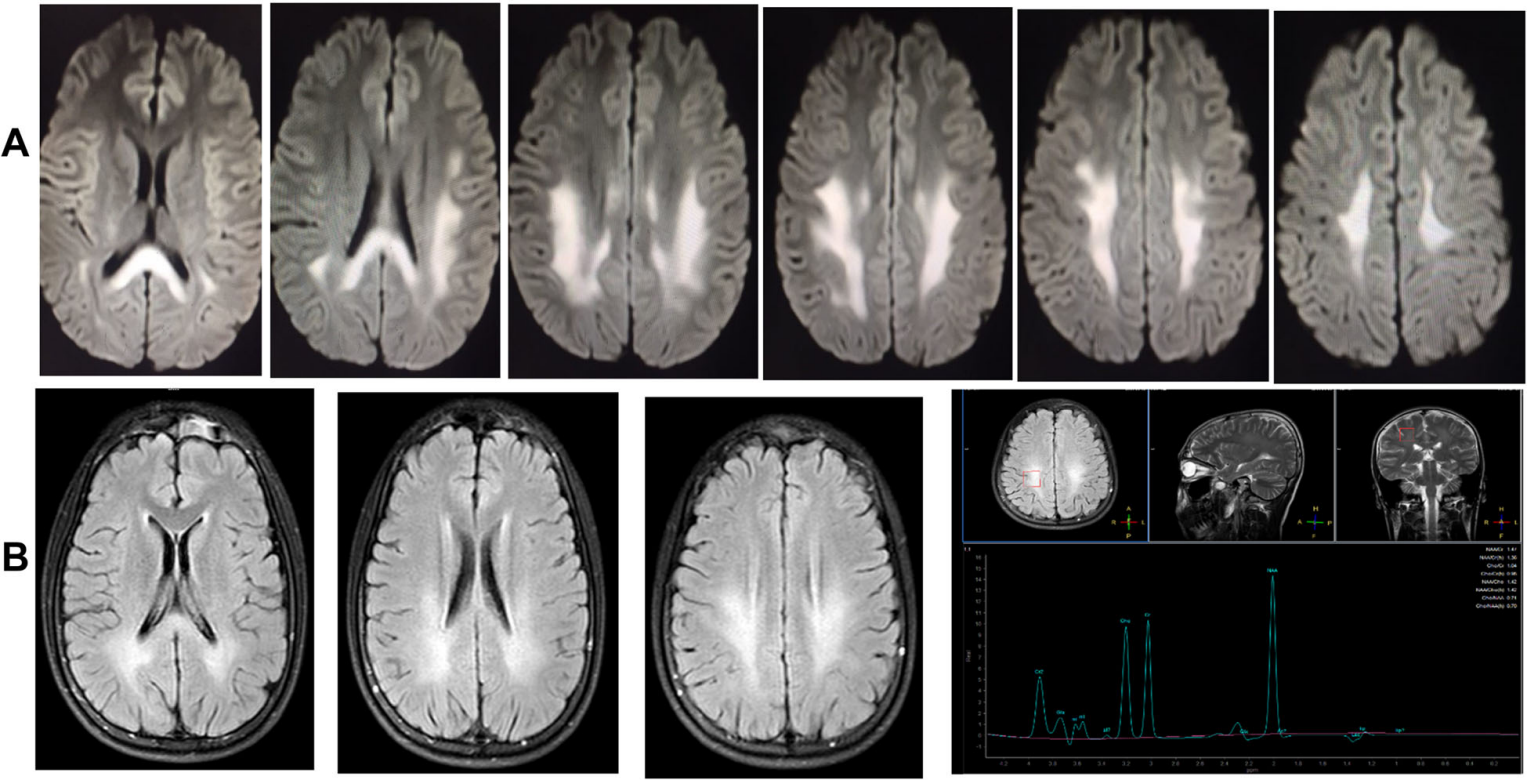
Brain magnetic resonance images of patient 1. a: MRI on 24 July 2018: Diffuse white matter lesions in posterior subcortical areas and the splenium of the corpus callosum. MRA was normal. b: MRI on 6 August 2018: the demyelinating changes of white matter had largely disappeared. MRS was normal
